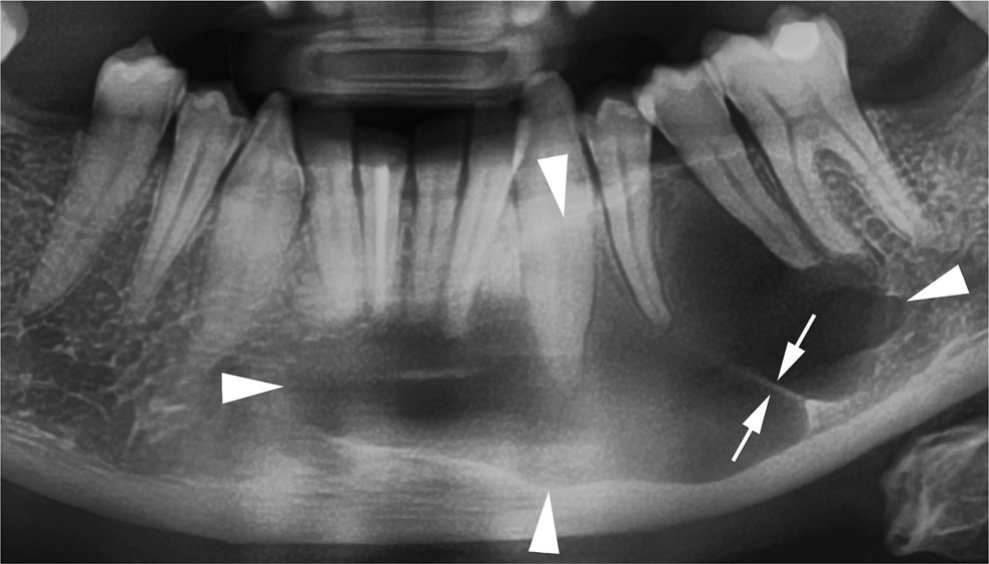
Cropped panoramic radiograph shows a large OKC with well-defined and lobulated margins (*arrowheads*) occupying the body of the mandible. Note an incomplete internal septum within the lesion (*opposing arrows*)
